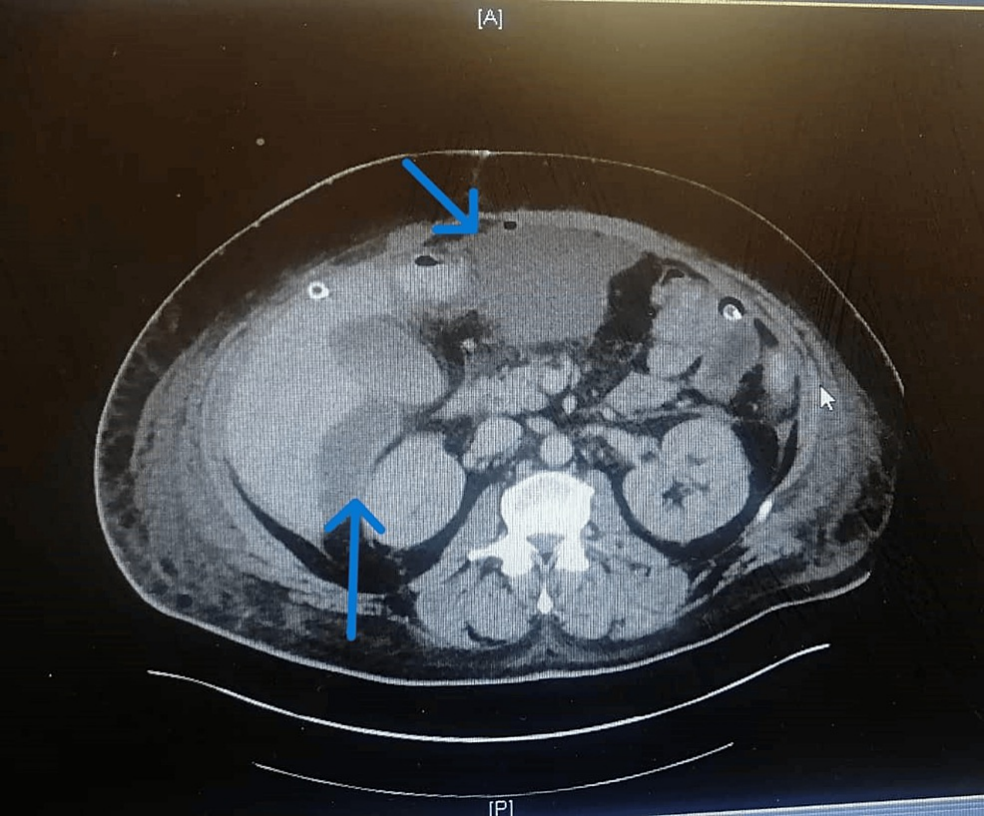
A follow-up CT of the abdomen and pelvis with oral and IV contrast showing sizable undrained collections anteriorly, in the subhepatic region, and at the left upper quadrant.
